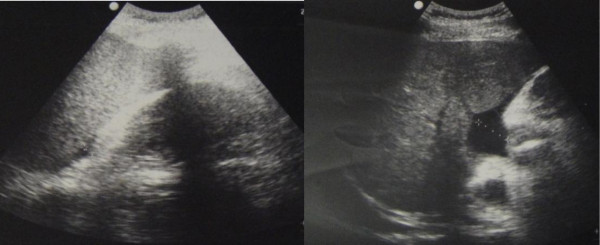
Moderate amounts of ascites were detected in case 1 after treatment with IL-11 by color doppler ultrasound: 2.1 cm in perihepatic region, 0.8 cm in perisplenic region, and 2.8 cm in the right lower abdomen.
